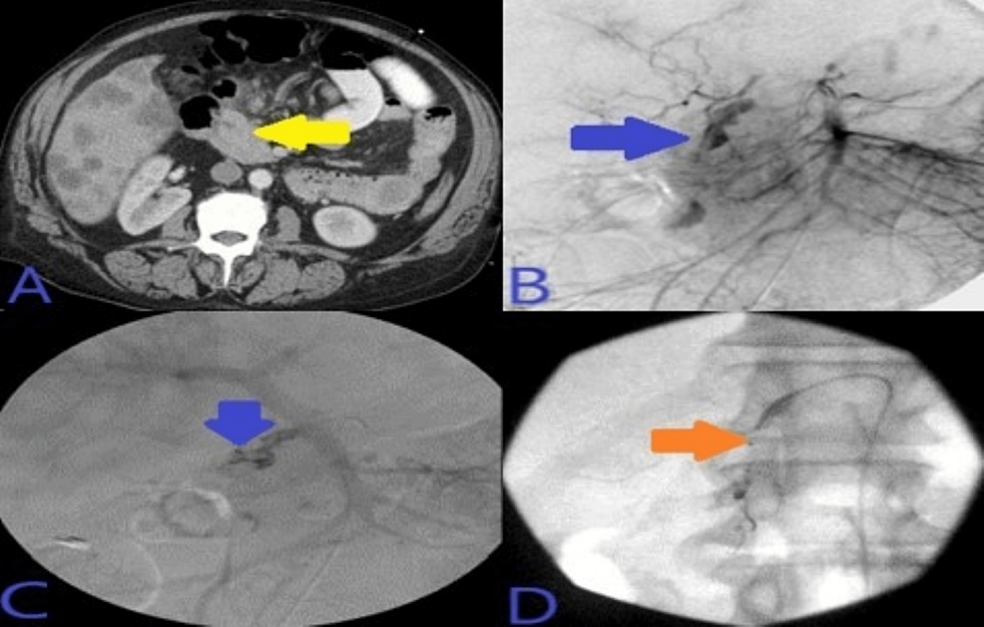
A: CT demonstrated hypertrophic submucosal arterioles along the duodenum compatible with oozing ulceration as seen on endoscopy. B: SMA angiogram demonstrating large active and delayed portal venous phase (image C). D: Post-embolization celiac angiogram demonstrated no evidence of active bleeding signs, and complete resolution of the above findings after deployment of two coils and one vascular plug in appropriate positions along the GDA. CT: computed tomography; SMA: superior mesenteric artery; GDA: gastroduodenal artery.
